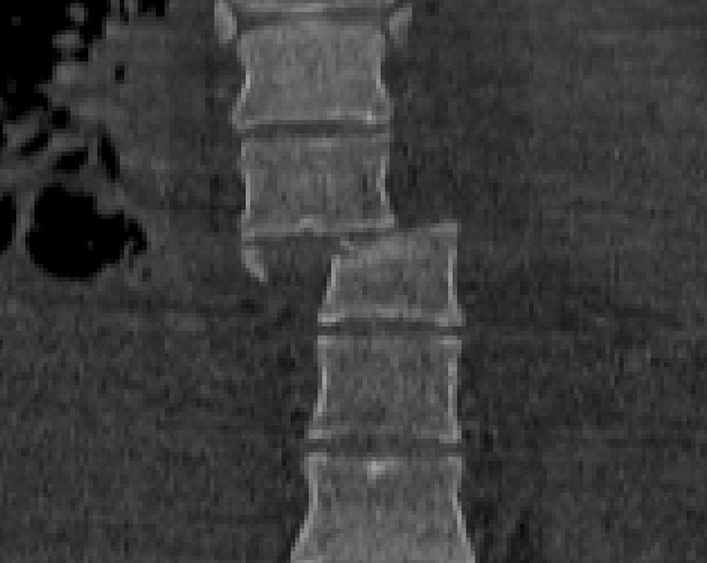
Rotational fracture-dislocation mechanism, CT scan, coronal view
